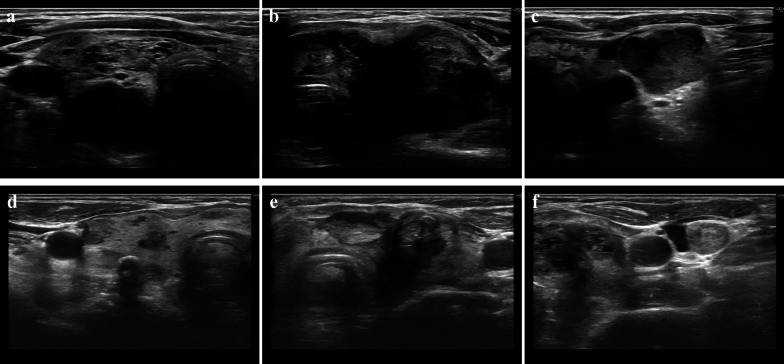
Ultrasonography before and after lenvatinib treatment. a–c Ultrasonography revealed a hypoechoic mass in the left lobe of the thyroid and lymph node metastases in the right paratracheal and left cervical regions. d–f A hypoechoic mass in the left lobe of the thyroid and lymph node metastases in the right paratracheal and left cervical regions shrank after lenvatinib treatment
